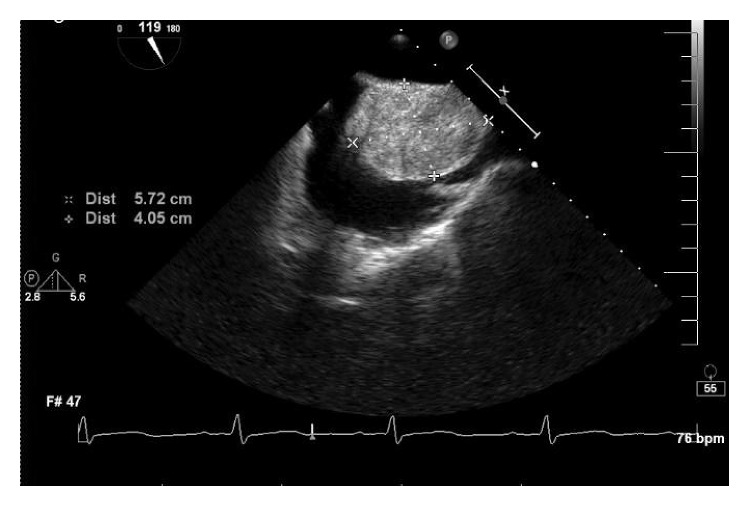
Transoesophageal echocardiogram showing the right atrial mass of 40 mm × 57 mm.
